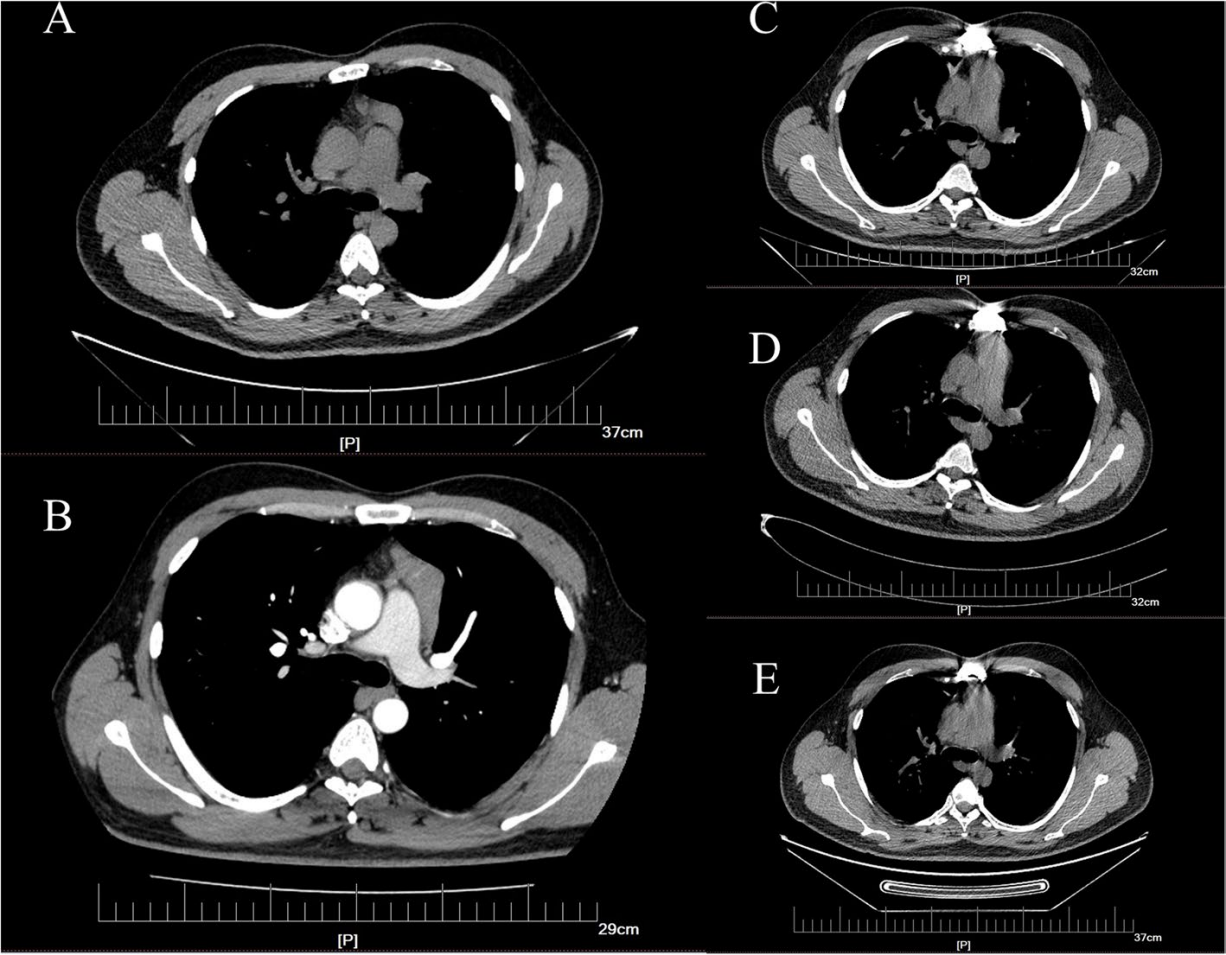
A The patient’s chest CT one year before admission, the size of the lesion was about 2.1*2.7 cm; B The patient’s arterial stage of chest enhancement CT on admission, with a lesion size approximately 5.3*2.2 cm; C The patient’s 3-month postoperative chest CT; D The patient’s 9-month postoperative chest CT; E The patient’s 2-year postoperative chest CT
